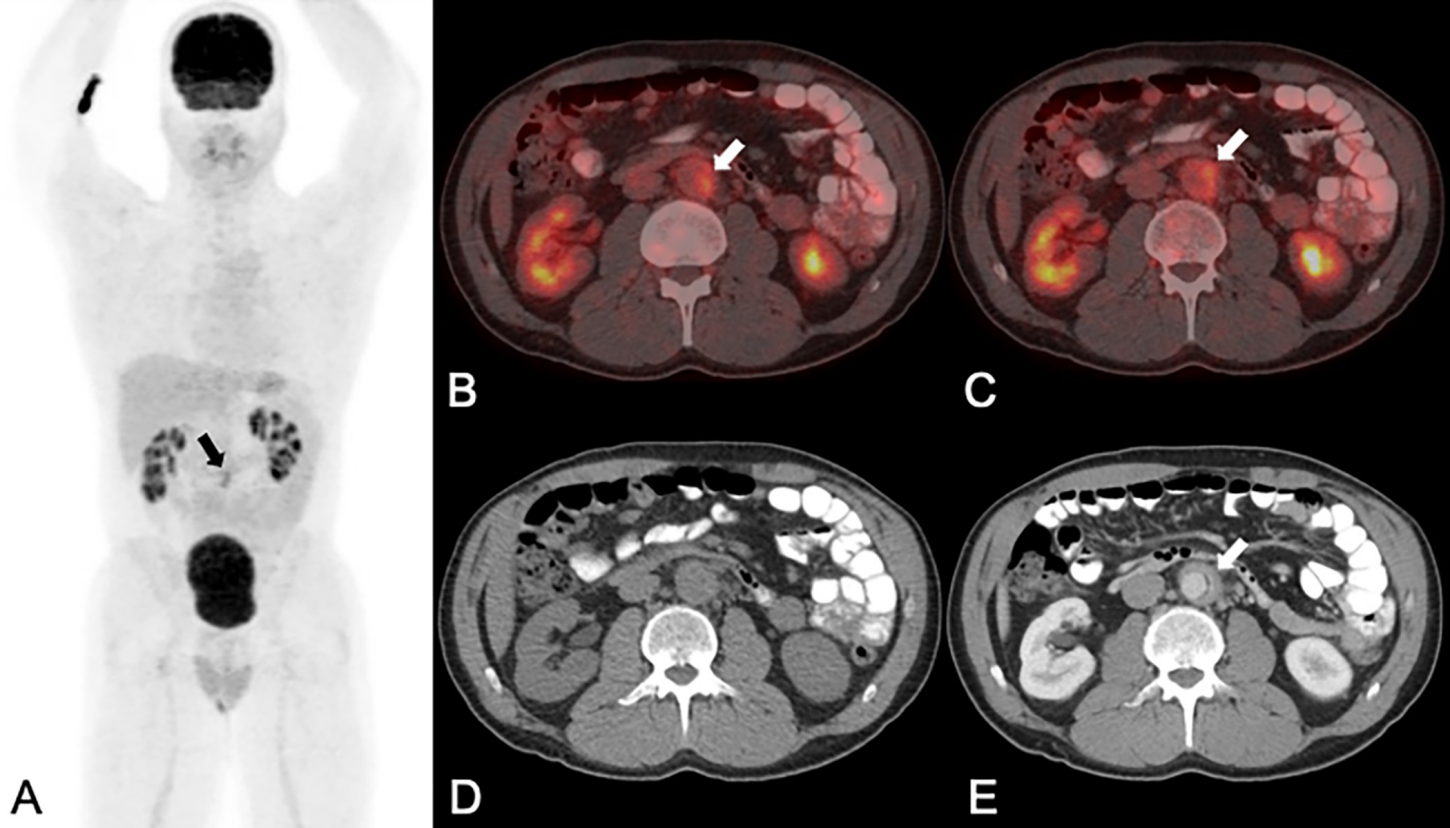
A 42-year-old man with chronic periaortitis, initially presenting with left-sided abdominal pain. PET/CT (A: maximum intensity projection of PET; B and C: fused PET/CT images (B) and contrast-enhanced CT (CE-CT: E) showed a 32 mm large abdominal aortic aneurysm (white arrows in B, C and E) with increased FDG uptake (SUVmax 3.5 in A-C) in the thickened (10 mm) aortic wall (white arrow in E). Using PET/CT images alone (A-D) both readers suspected an infectious/mycotic aneurysm (notably, wall thickening may not be clearly delineated on the non-enhanced CT part of the PET/CT in D, but only on the CE-CT in E). With CE-CT (E) and with combined imaging (not shown) both readers correctly diagnosed an inflammatory aneurysm. The patient was subsequently treated with steroid therapy for four months and no vascular intervention was performed. The latest follow-up imaging eight years after the initial diagnosis (not shown), showed no residual wall thickening in the normal sized (18 mm) abdominal aorta and the patient had no abdominal symptoms.
